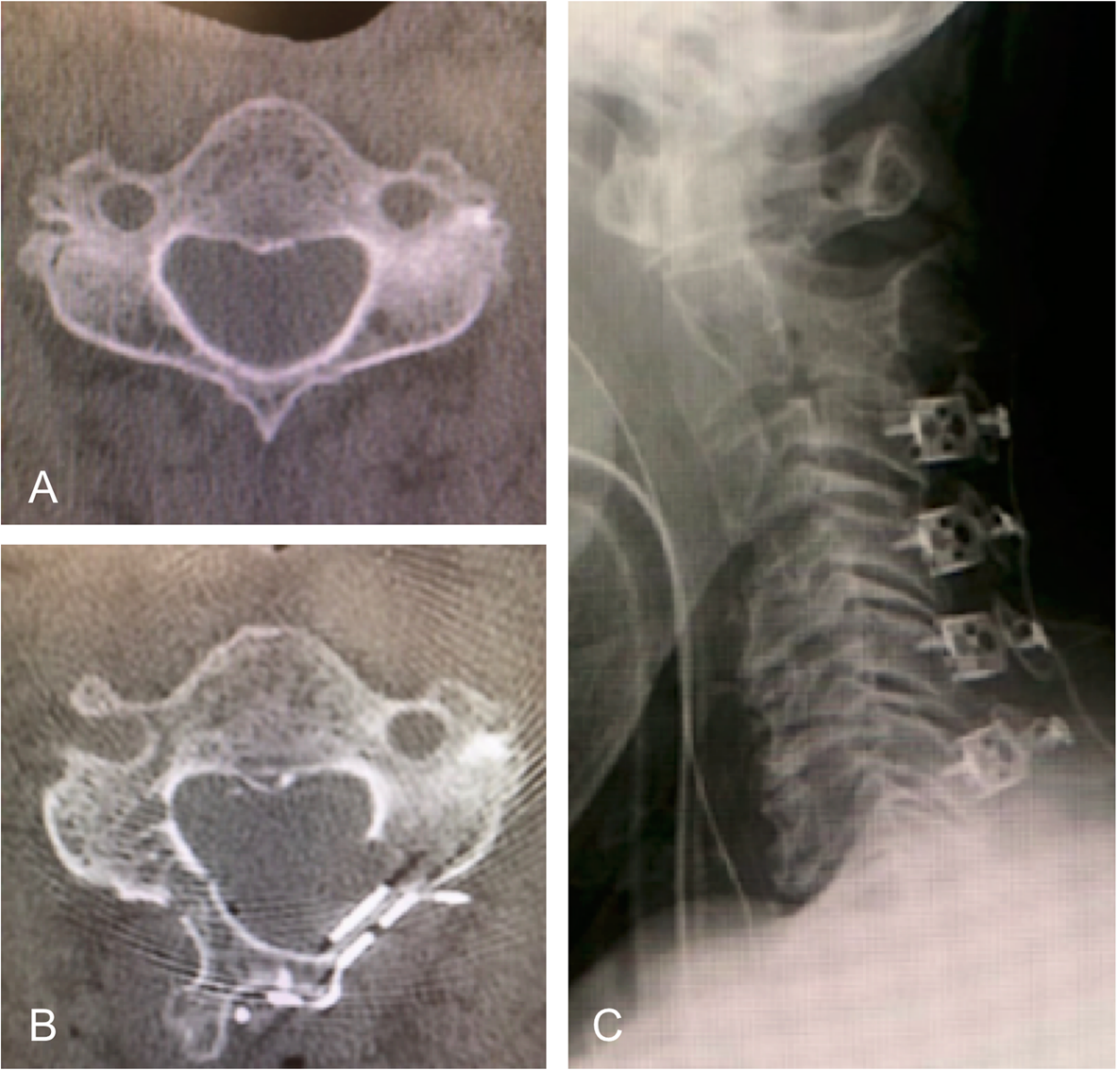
Example of a case of open door laminoplasty using titanium plate spacers. a Preoperative computed tomography image. b Postoperative computed tomography image. c Postoperative radiograph
